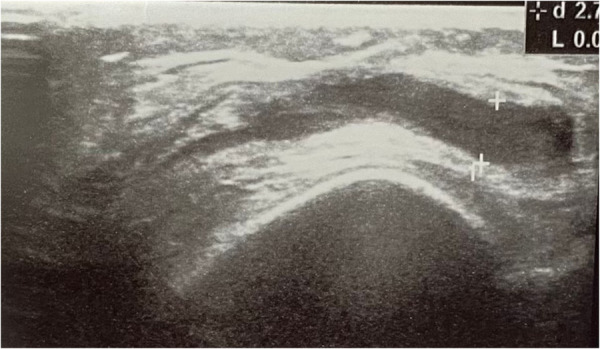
Knee ultrasound demonstrating periarticular hypoechoic synovial thickening measuring about 2.5 × 0.5 cm, suggestive of synovitis.
